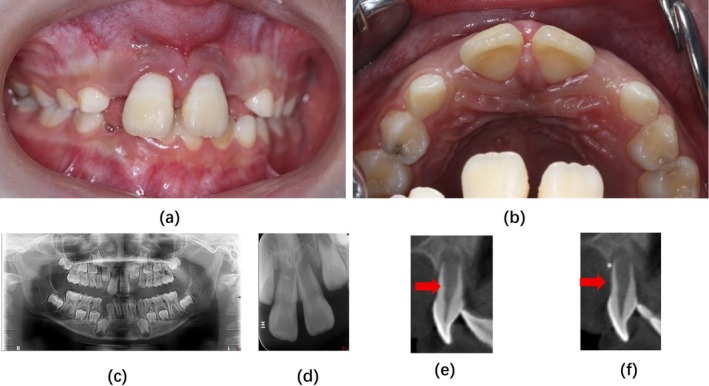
Pre‐surgical intraoral and radiograph views. (a) Labial view showed swelling and misalignment of teeth 11 and 21; (b) occlusion view showed swelling around teeth #11 and #21; (c–f) pre‐surgical radiograph showed mixed dentition, with high‐density shadows on the labial aspect of 11, teeth #11 and #21 and an arch‐like bone defect around the root.
